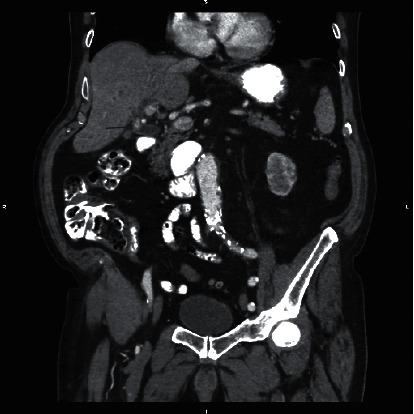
Coronal CT of the abdomen with contrast demonstrates 11 mm hyperdensity likely representing a calculus at the origin of the common bile duct (arrow).
